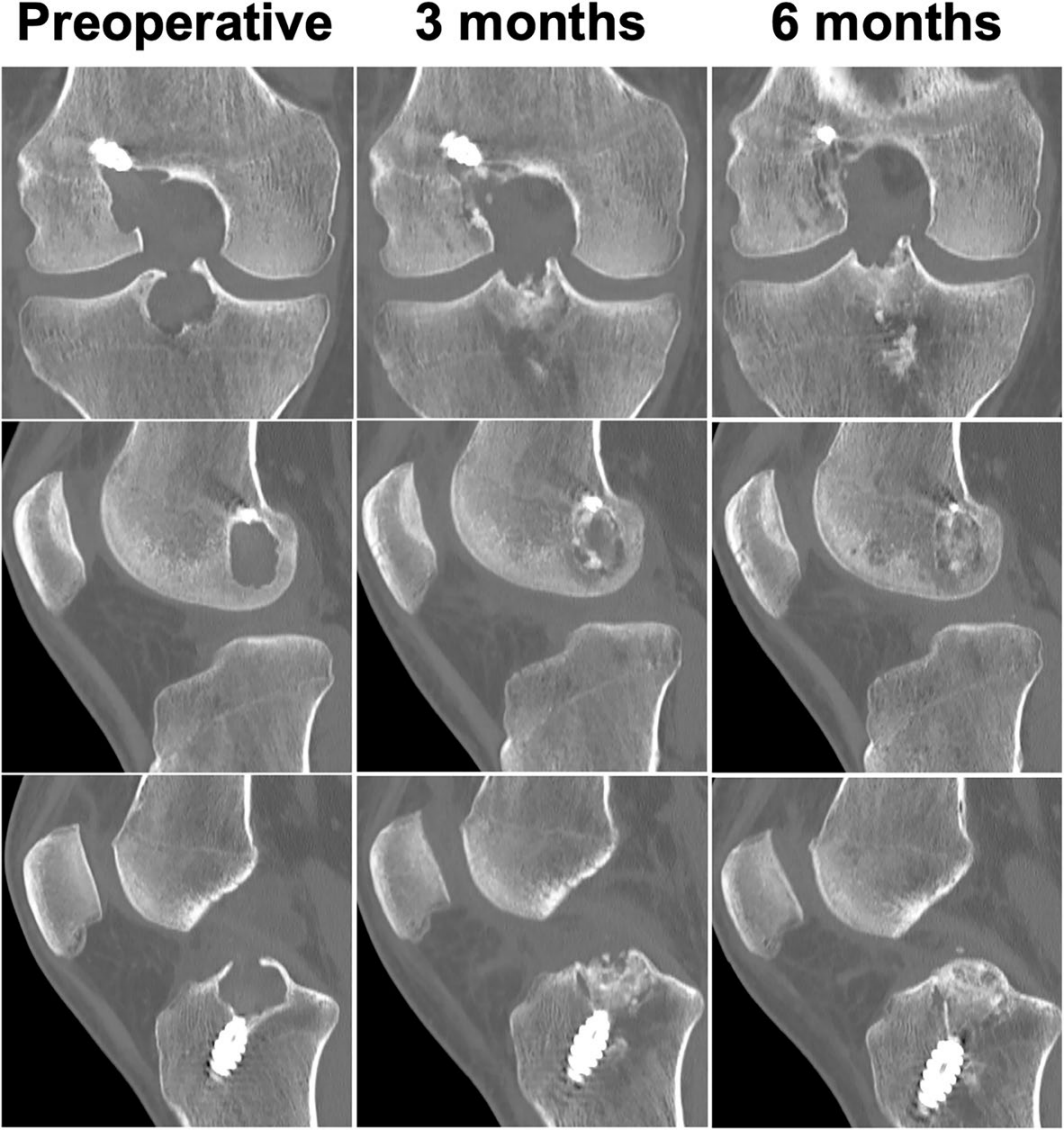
Comparison of preoperative CT scans (left column) with scans acquired at 3 (middle column) and 6 months (right column) after index surgery showing progressive integration of the autologous bone graft in both femoral and tibial tunnels without signs of resorption
